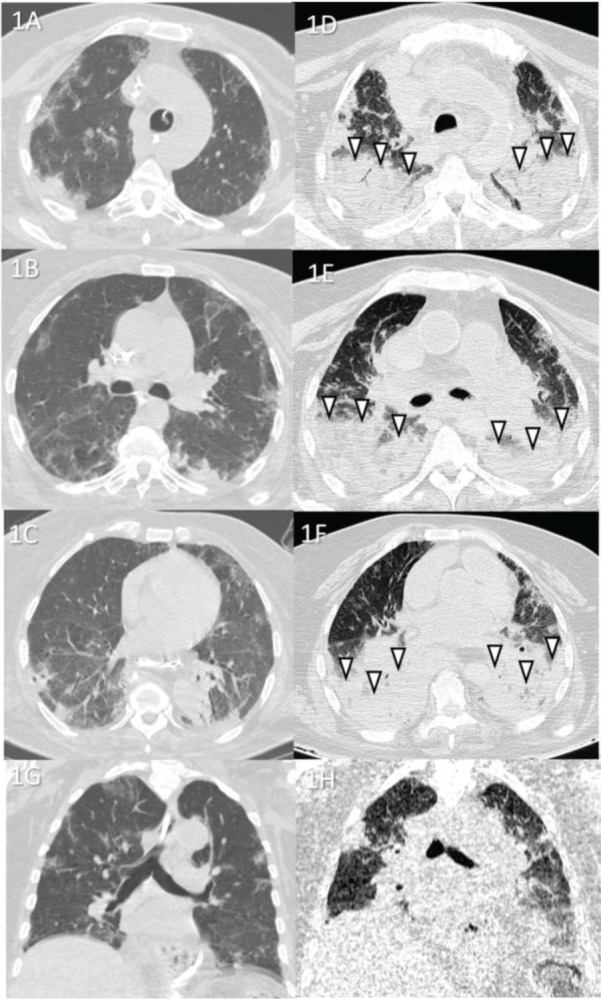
Case 1: Premortem axial chest computed tomography (CT) of the upper (A), mid (B), and inferior (C) thirds of the lungs obtained 2 days before death showing peripheral ground-glass opacities, slight consolidations, and interlobular septal thickening. Postmortem axial chest CT of the upper (E), mid (F), and inferior (G) thirds of the lungs obtained 4 h 47 min after death showing opacities larger than those on premortem CT, thus demonstrating the progression of the disease until death. Postmortem posterior “horizontal level forming” consolidations because of hypostasis (white arrowheads) are also observed, which limited analysis of the posterior lungs in this case (limitation of the method). Images D and H show pre- and postmortem coronal reformats, respectively.
